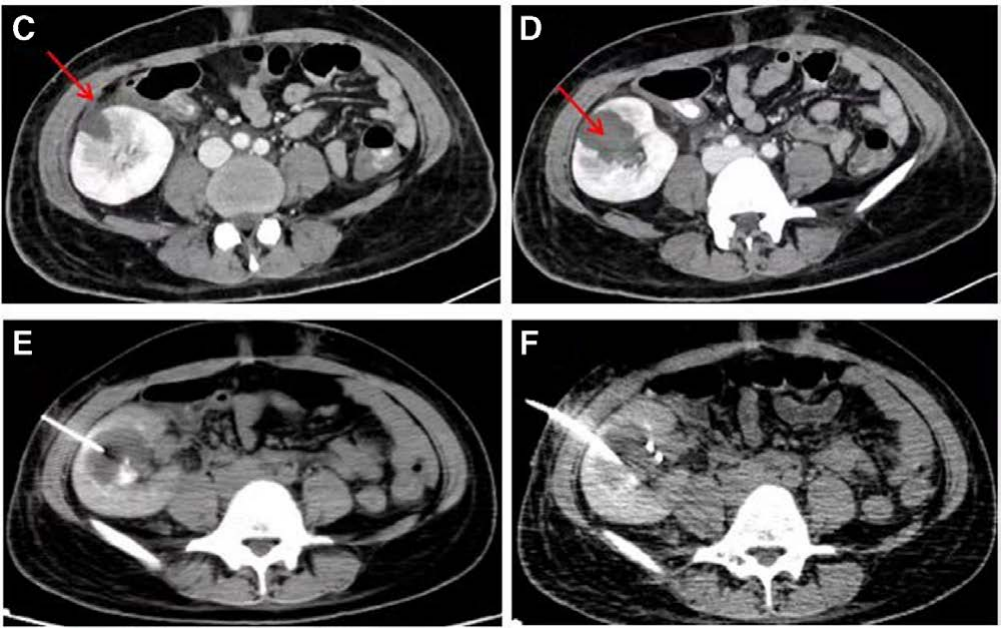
(C–F) Contrast-enhanced CT revealed a lesion with no enhancement indicative of abscess formation, which involved renal parenchyma and medulla and ruptured into perirenal space (arrow). CT = computed tomography.
